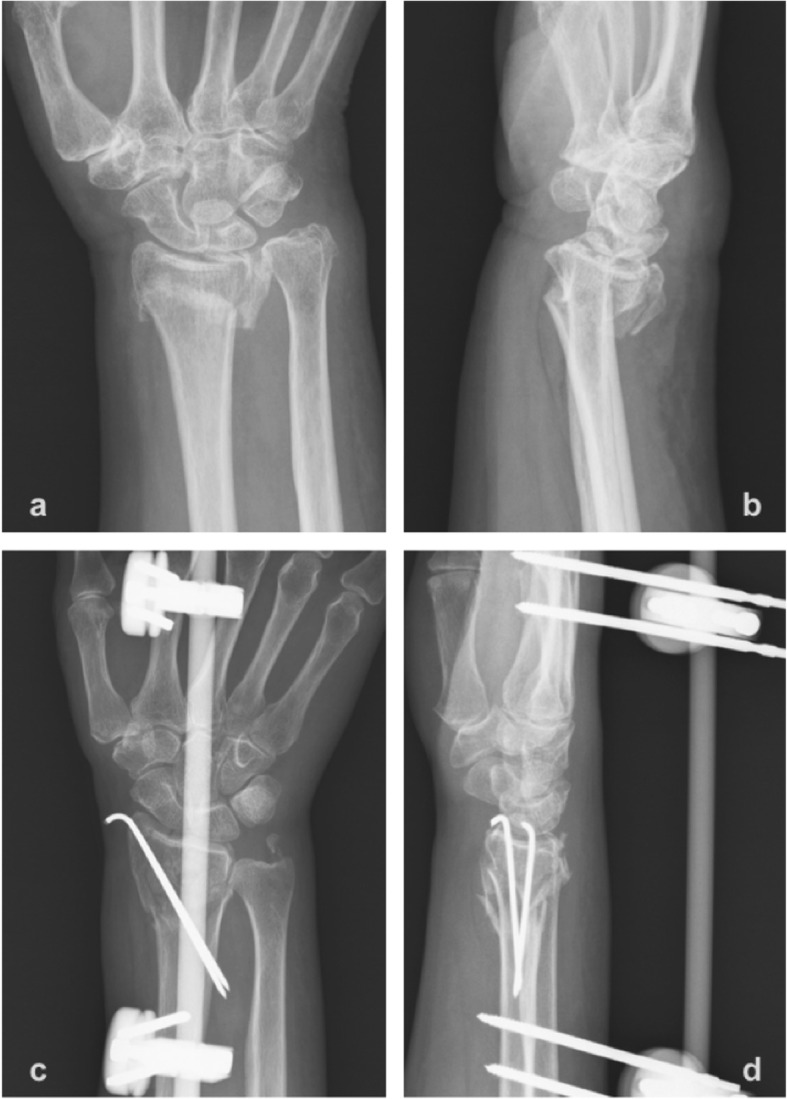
a, b; The anteroposterior and lateral plain films showed an intra-articular comminuted distal radius fracture with dorsal displacement. c, d; Immediate postoperative anteroposterior and lateral plain films presented after adequate reduction, external fixator and K-pins fixation
